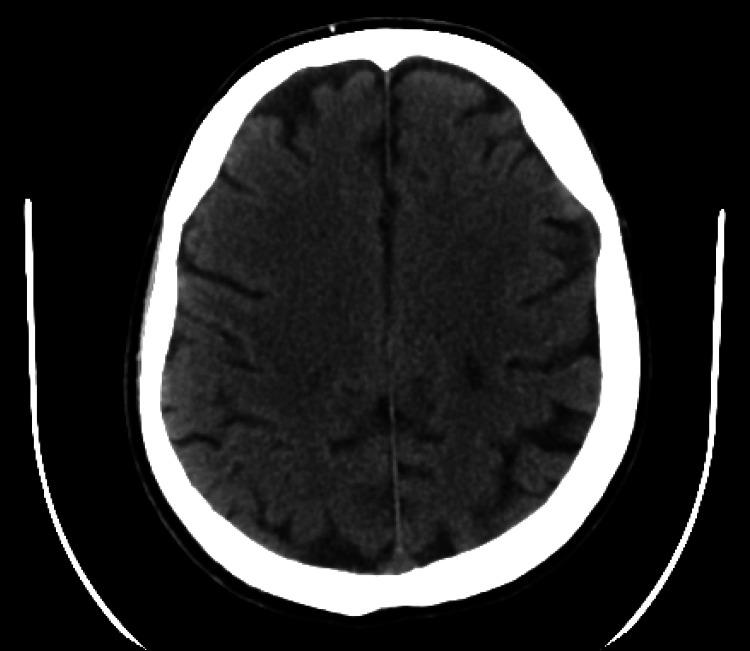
CT scan of the brain showing a 10 mm hypodense image in the posterior part of the semi-oval lobe consistent with a subacute ischemic process.
